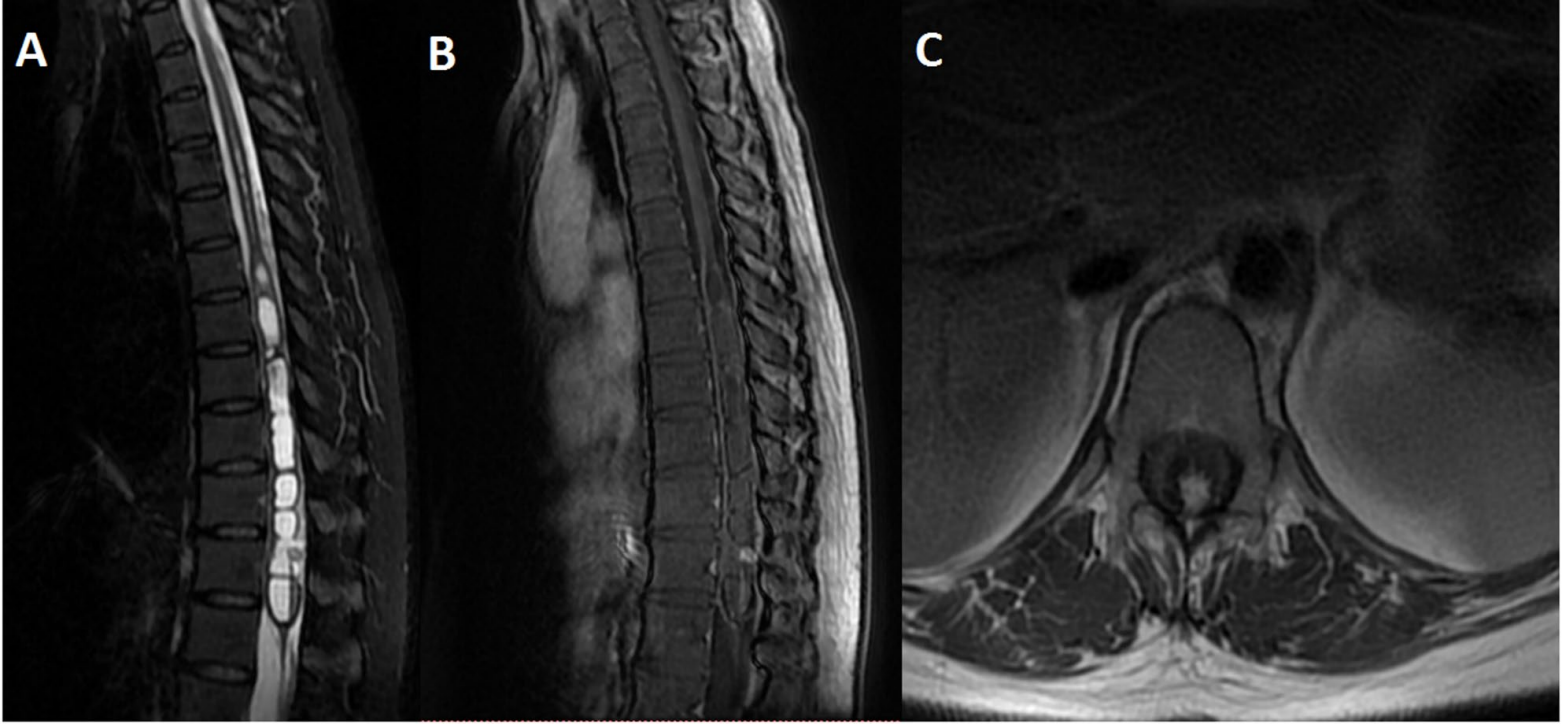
MRI showed a well-defined small intramedullary mass. The lesion appeared hypointense on sagittal T2WI (A) and axial T2WI (C), a nodular enhancement after gadolinium infusion (B). Syrinx could also be seen above and below the nodular (A)
